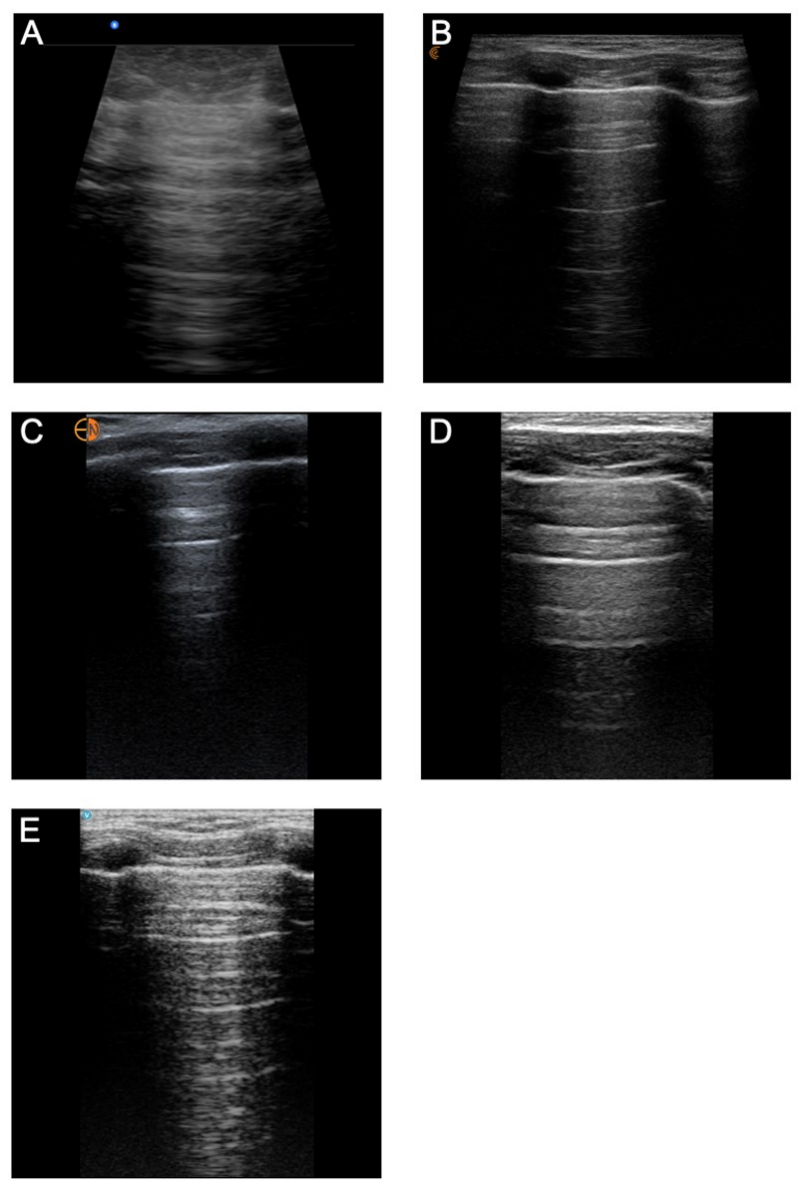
Superficial Neck Views acquired from the same pediatric model using six handheld ultrasound devices: (A) Butterfly iQ+™, (B) Clarius®, (C) Kosmos™, (D) Lumify™, (E) Vscan Air™.
